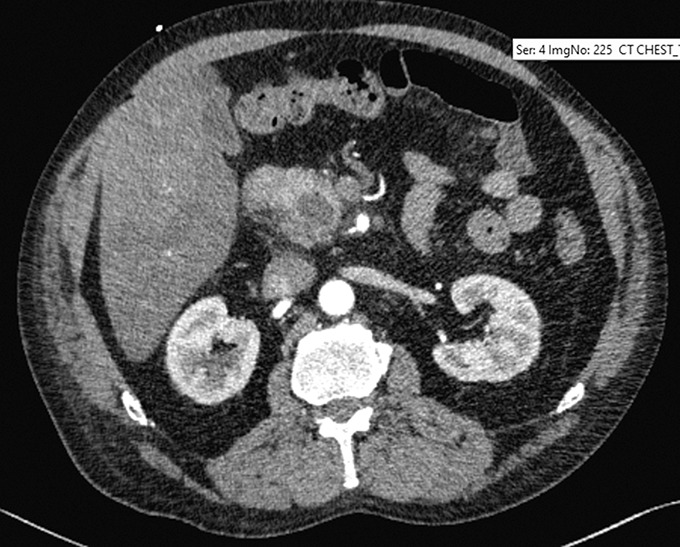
Computed tomography abdomen showing peripancreatic fat stranding with a hypodense area in the pancreatic head/uncinate.
